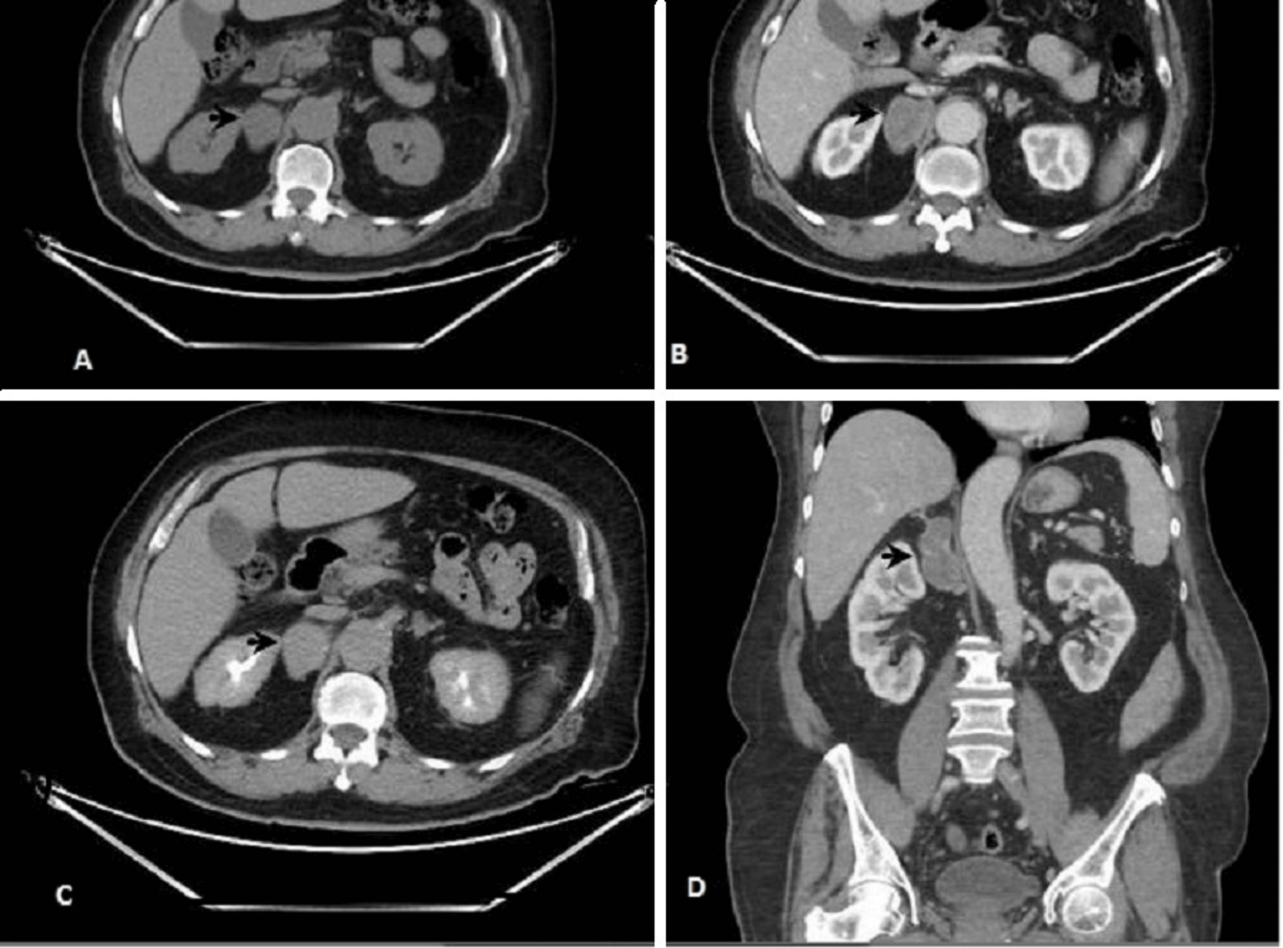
CT with contrast study. (A) Precontrast phase; mass in the right adrenal gland, 30 HU in noncontrast image; (B and D) mass enhancing to 55 HU in the portal venous phase. The mass is clearly localized to the right adrenal region, separate from the kidney. No significant invasion of adjacent structures, supporting its benign nature. (C) Persistent enhancement of the lesion to 65 HU in the delayed phase. The lesion exhibits mild progressive enhancement, a characteristic feature of schwannomas due to their fibrous nature. CT: computed tomography
